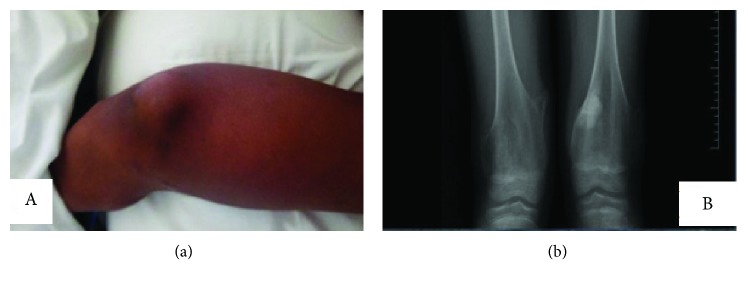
(a) marked swelling and induration of the left distal femur and (b) X-ray shows bone outgrowths (exostoses) on the medial and lateral aspects of the distal femur bilaterally and left metaphyseal widening (AP view).
